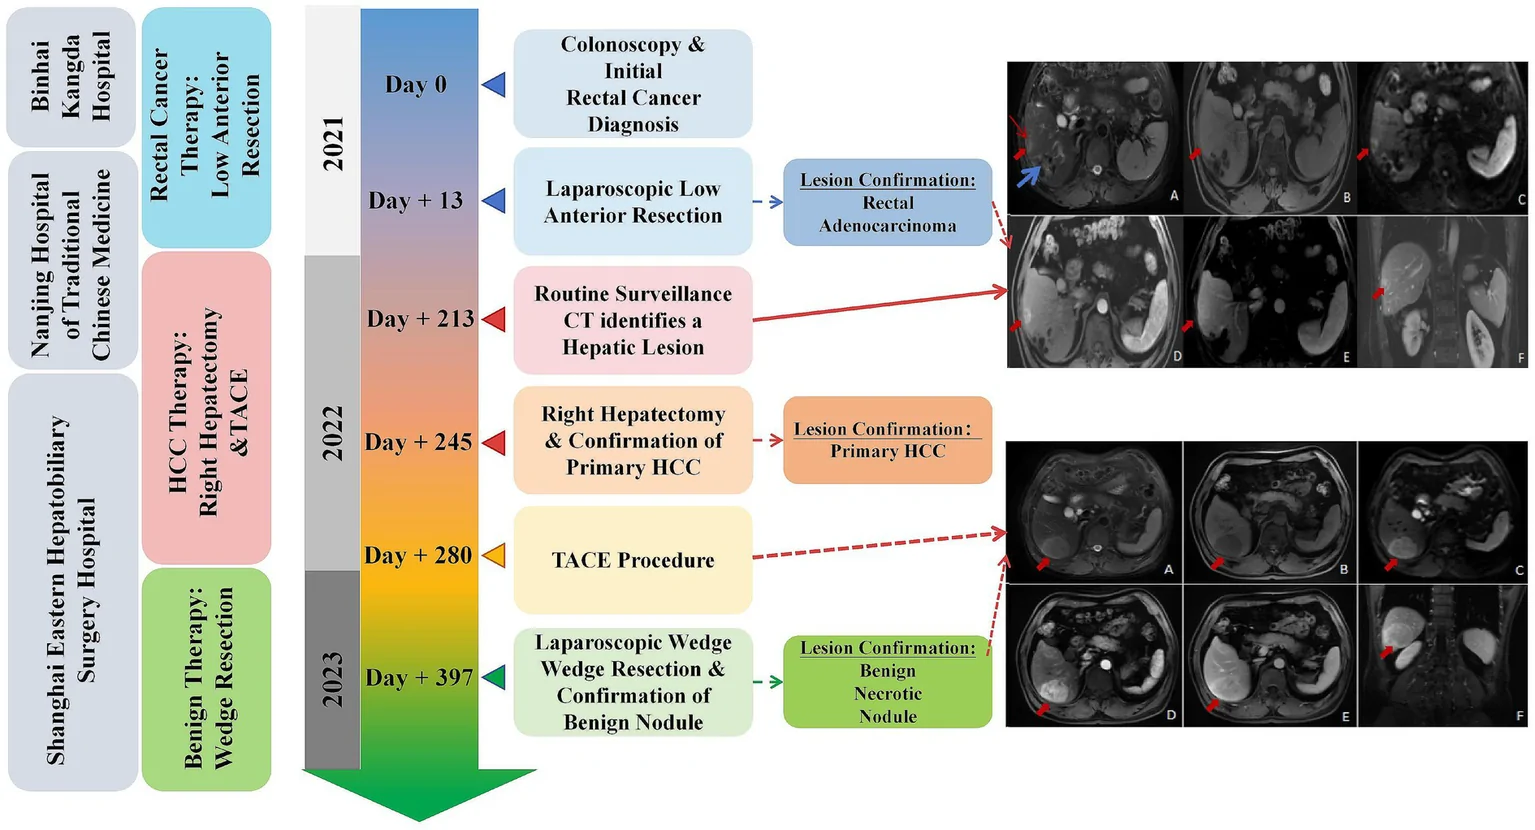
Timeline of the clinical course for the patient with rectal adenocarcinoma, followed by primary hepatocellular carcinoma (HCC) and a benign necrotic liver nodule. Date markers are in relative days (Day 0 = initial rectal cancer diagnosis). Right selected MRI frames. Figures 2A–D shows characteristic findings of primary HCC (Day +213). Figures 5A–D shows characteristic findings of a benign necrotic hepatic nodule (Day +397). (red arrows point to the lesion).
